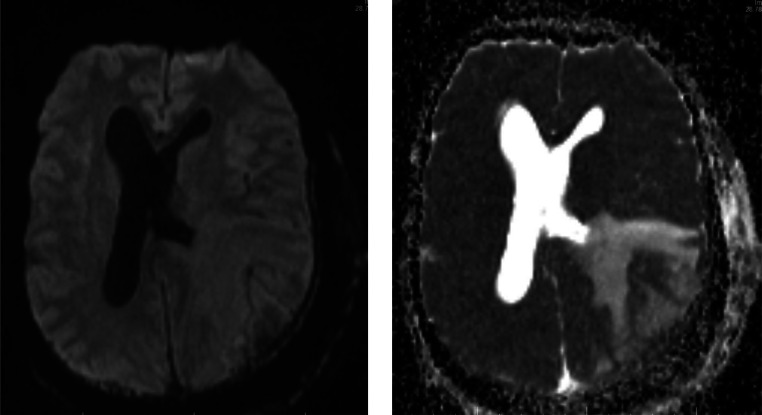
A&B DWI and ADC map show no diffusion restrictions areas.
